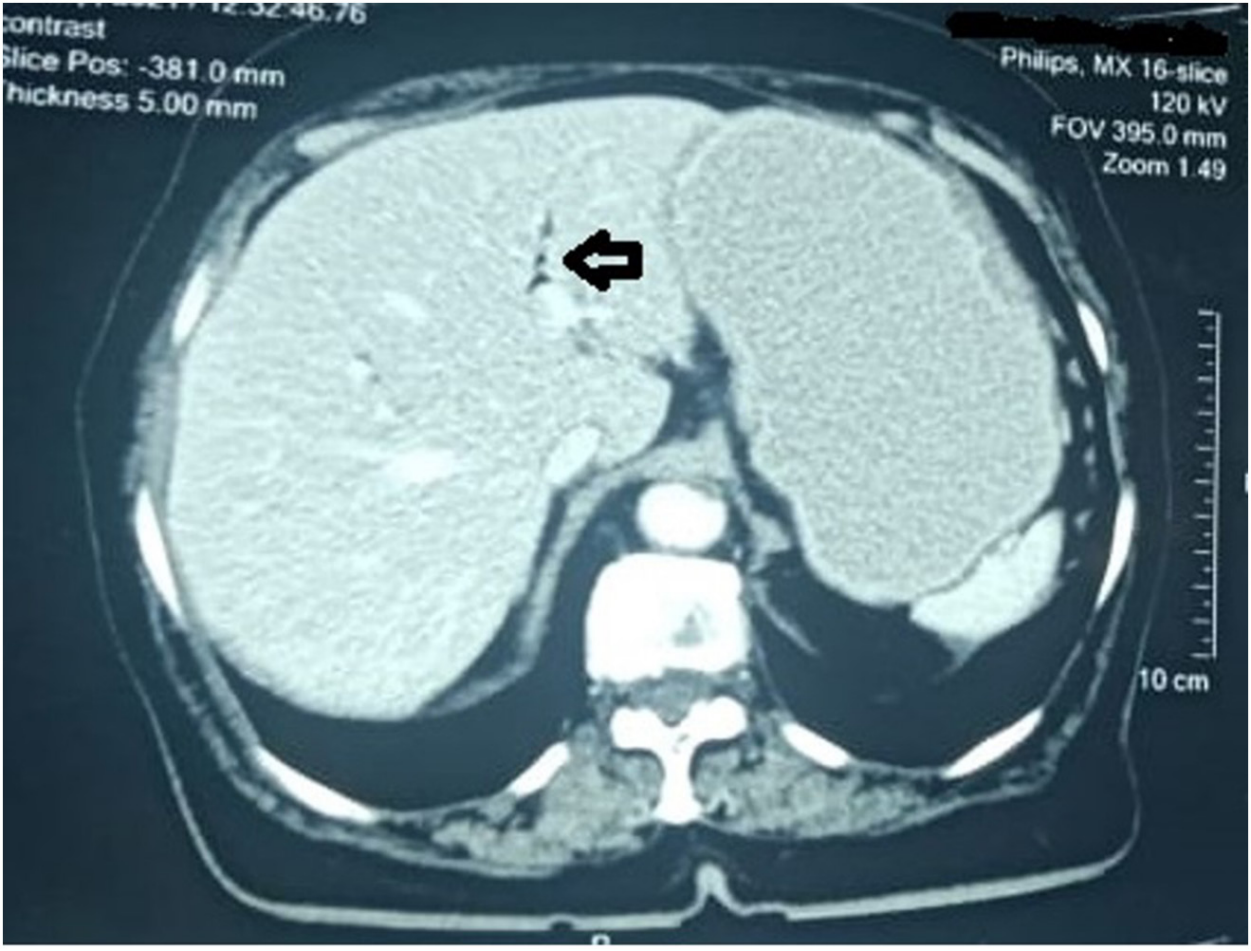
Contrast‐enhanced CT abdomen showing Rigler's triad: a distended stomach secondary to obstruction, air within the biliary channels (black arrow).
